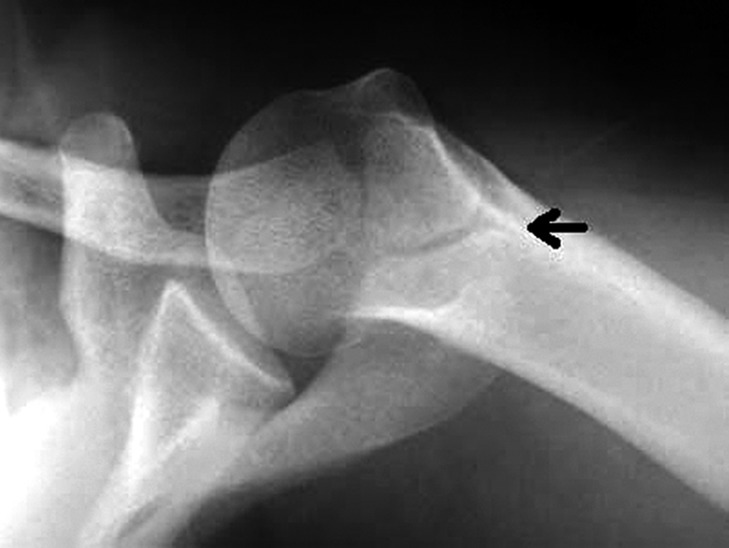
Axillary lateral radiograph demonstrating a meso‐acromion (arrow)^28^.
